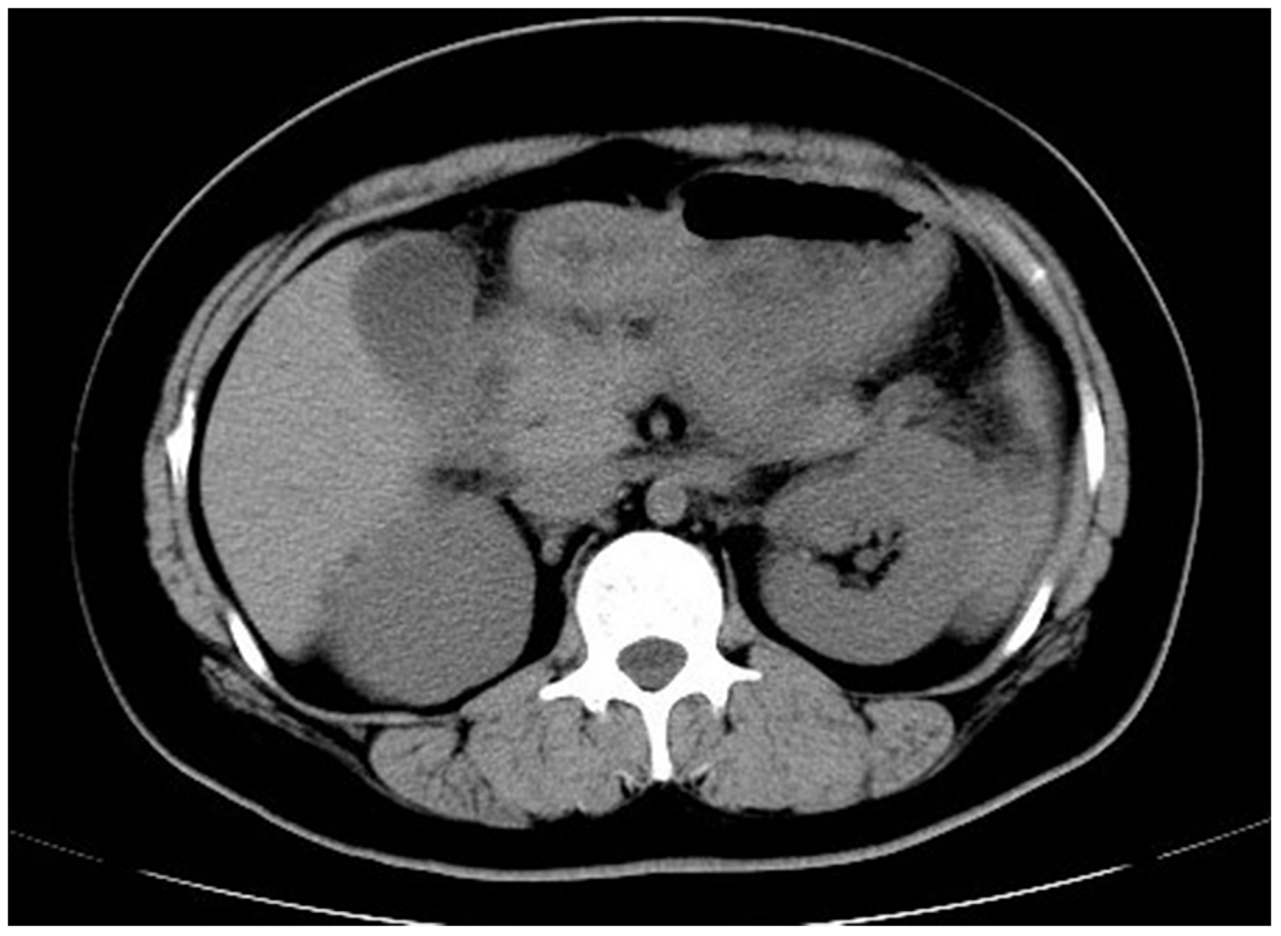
Abdominal computed tomography A typical computed tomography manifestation of enlarged pancreas with blurred outline, disappearance of peripancreatic space and thickened left renal fascia was highly suggestive of acute pancreatitis.
